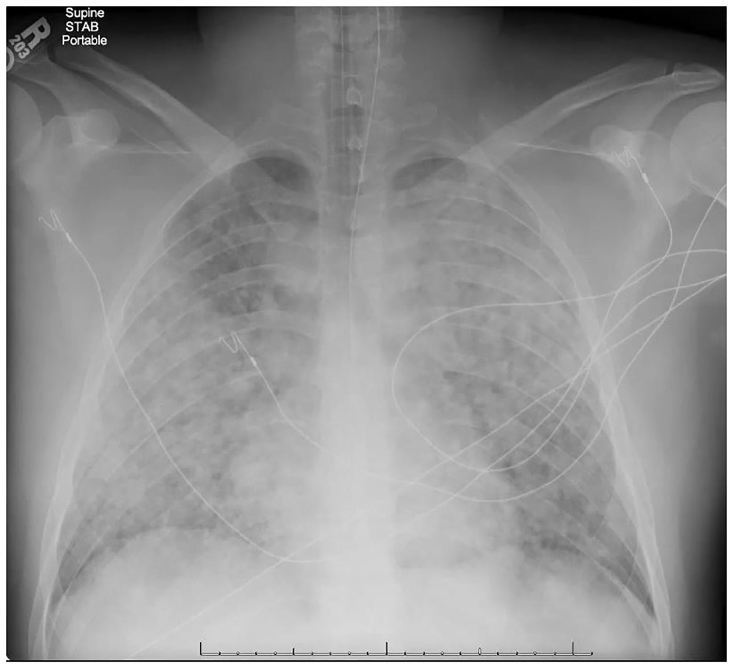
Anteroposterior chest radiograph taken in the emergency department showing bilateral, diffuse pulmonary opacities consistent with the acute respiratory distress syndrome.
